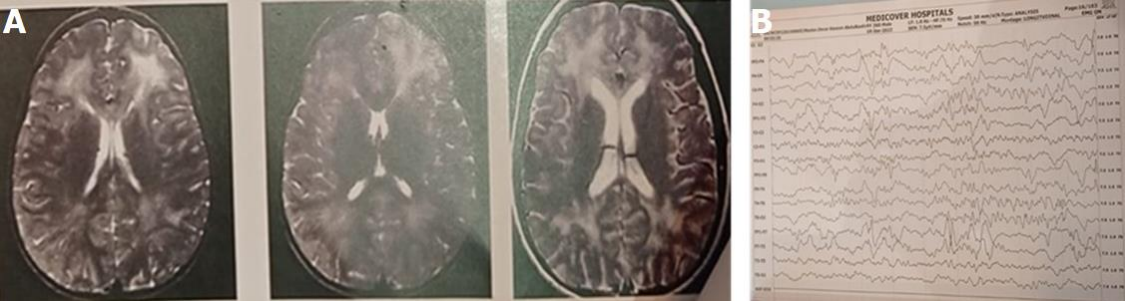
longitudinal bipolar montage showing periodic slow waves also known as the radermecker; A) Magnetic resonance imaging (MRI) demonstrating high signal intensity on T2 and FLAIR MRI in periventricular and subcortical white matter; B) EEG showing focal seizure with generalization epileptic form, suggesting encephalopathy as seen with periodic slow waves
